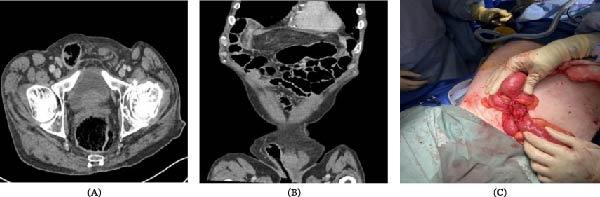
(A) Axial and (B) coronal CT images of the abdomen and pelvis demonstrating a right inguinal hernia containing small bowel loops, with associated inflammatory changes and gas tracking into the scrotal sac. (C) Intraoperative view showing an incarcerated and perforated Richter‐type hernia at the internal inguinal ring, involving the antimesenteric border of the terminal ileum.
